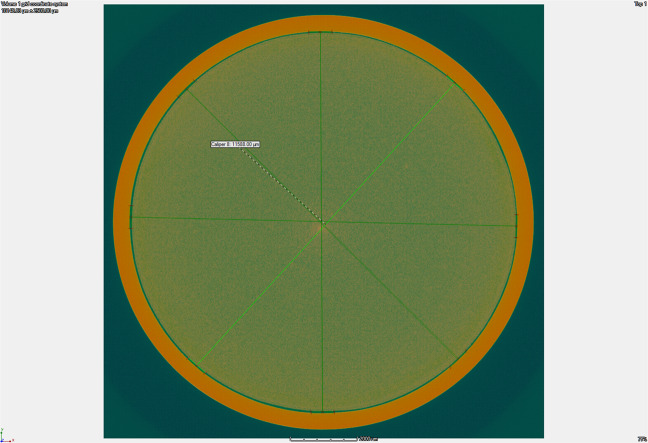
A typical tube cross-section image. X-ray computer tomography image of a tube cross-section.
